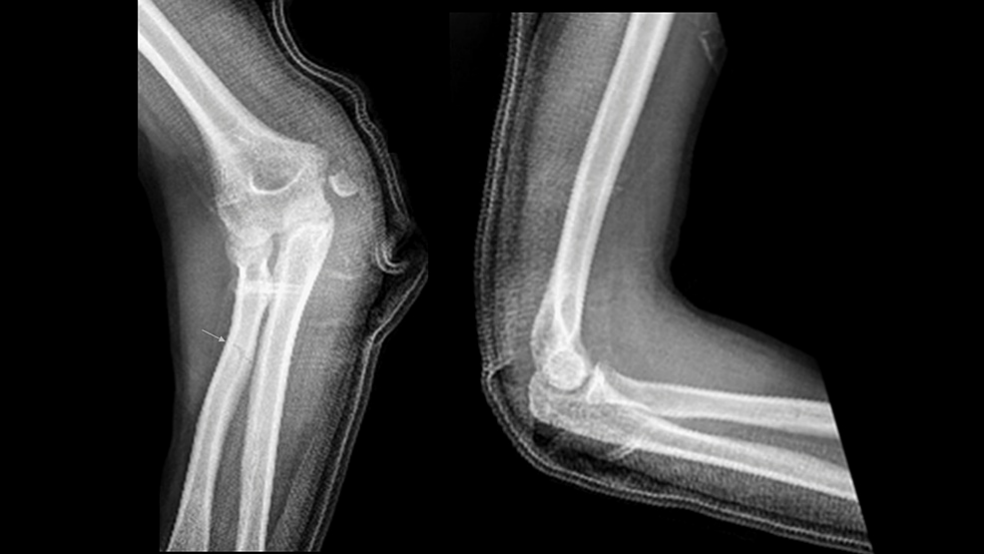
Radiographs of the elbow after elbow reduction showing displaced fracture of the medial epicondyle and non-displaced fracture of the radial shaft (arrow).
